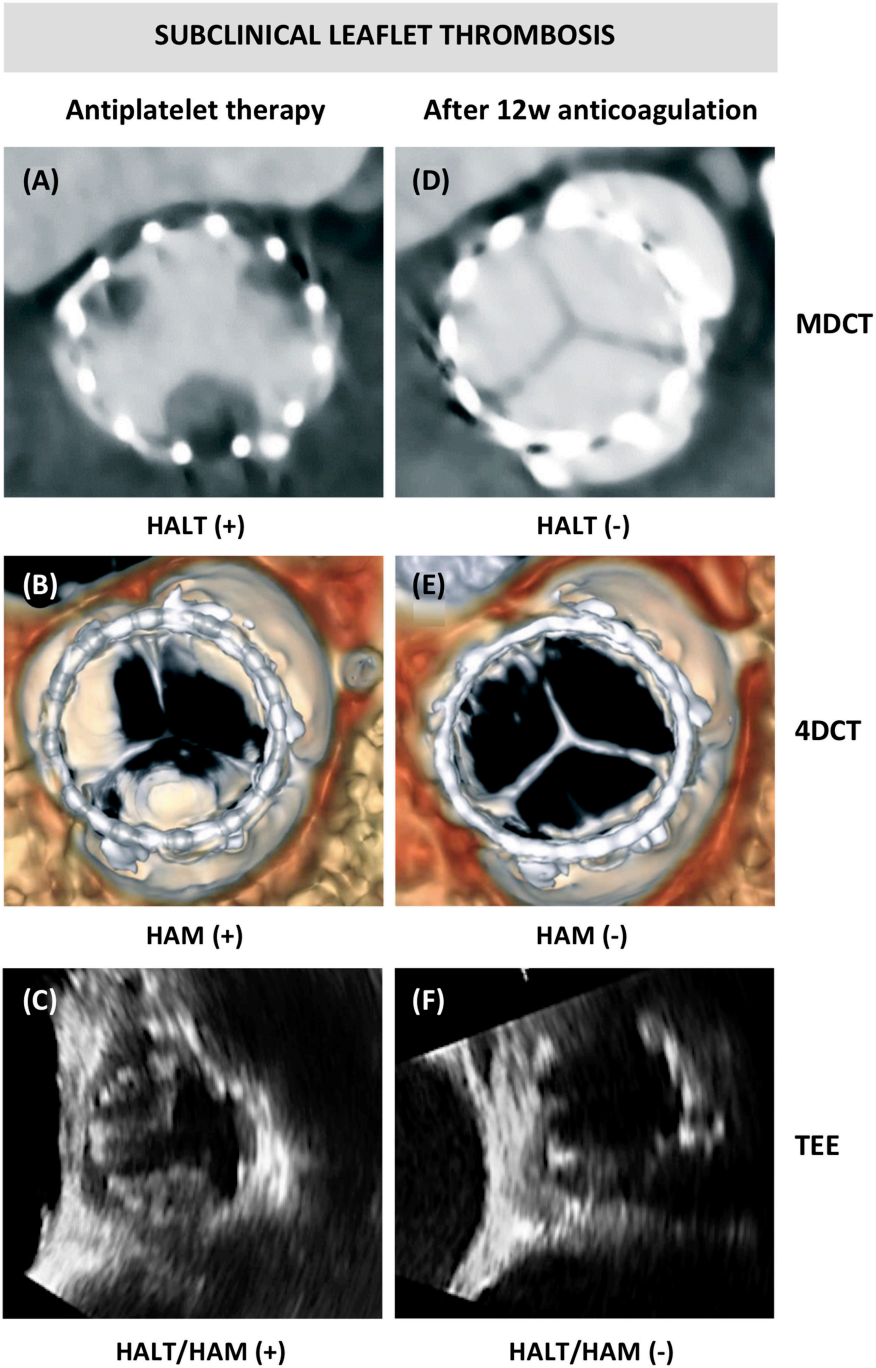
Subclinical leaflet thrombosis. (A) Computed tomography (CT) images showing hypoattenuating leaflet thickening (HALT) at the base of all three bioprosthetic leaflets, (B) with hypoattenuation affecting motion (HAM) visible in systole in the volume-rendered 4DCT images; and (C) transesophageal echocardiography (TEE) confirming reduced leaflet motion of two leaflets. (D–F) Resolution of HALT and HAM after 3 months of anticoagulation treatment.
